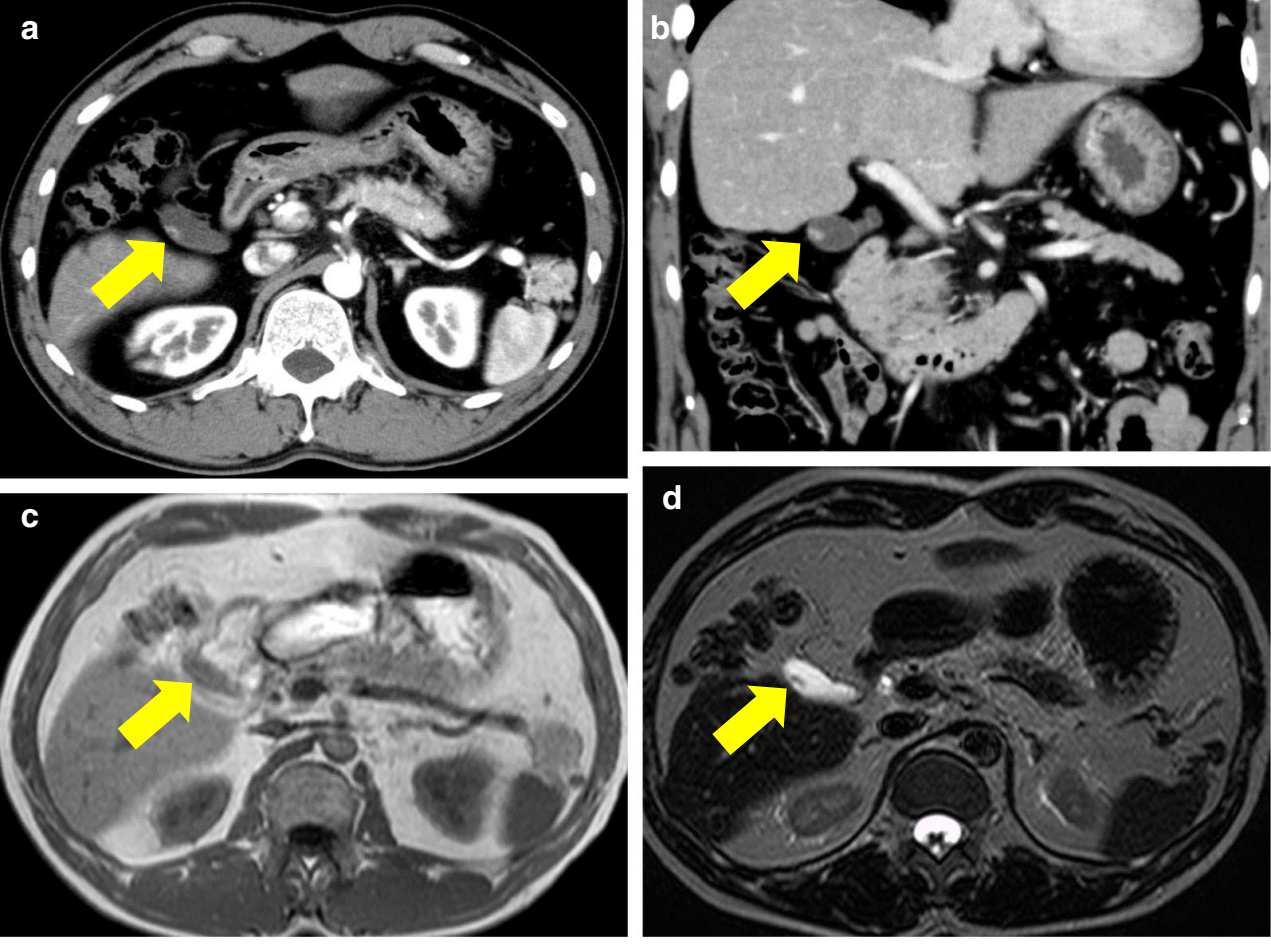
Contrast-enhanced computed tomography of the abdomen showed an enhanced polyp in the a axial and b coronal sections. c Axial T1-weighted magnetic resonance imaging showed that the polyp was isointense. d T2-weighted magnetic resonance imaging showed that the polyp was of low intensity
